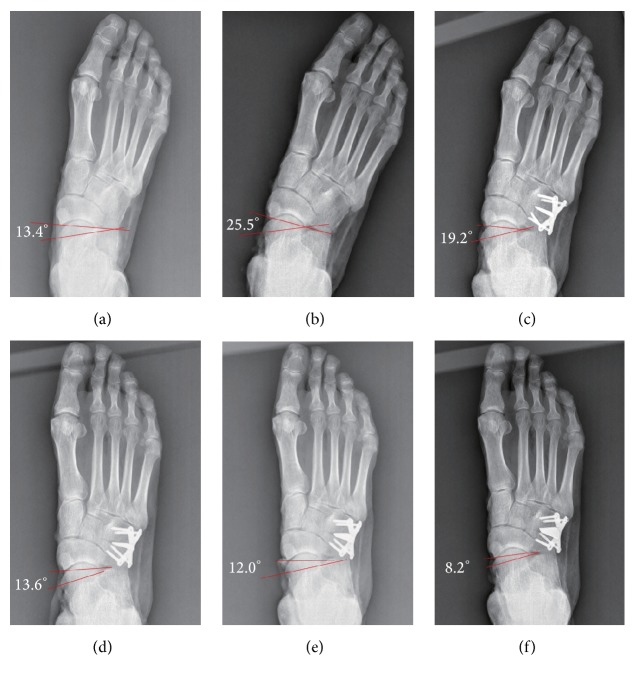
Anteroposterior radiographs of six conditions: (a) intact foot, (b) flatfoot, and (c–f) lengthening of lateral column with cuboid osteotomy (2 mm, 3 mm, 4 mm, and 5 mm).
